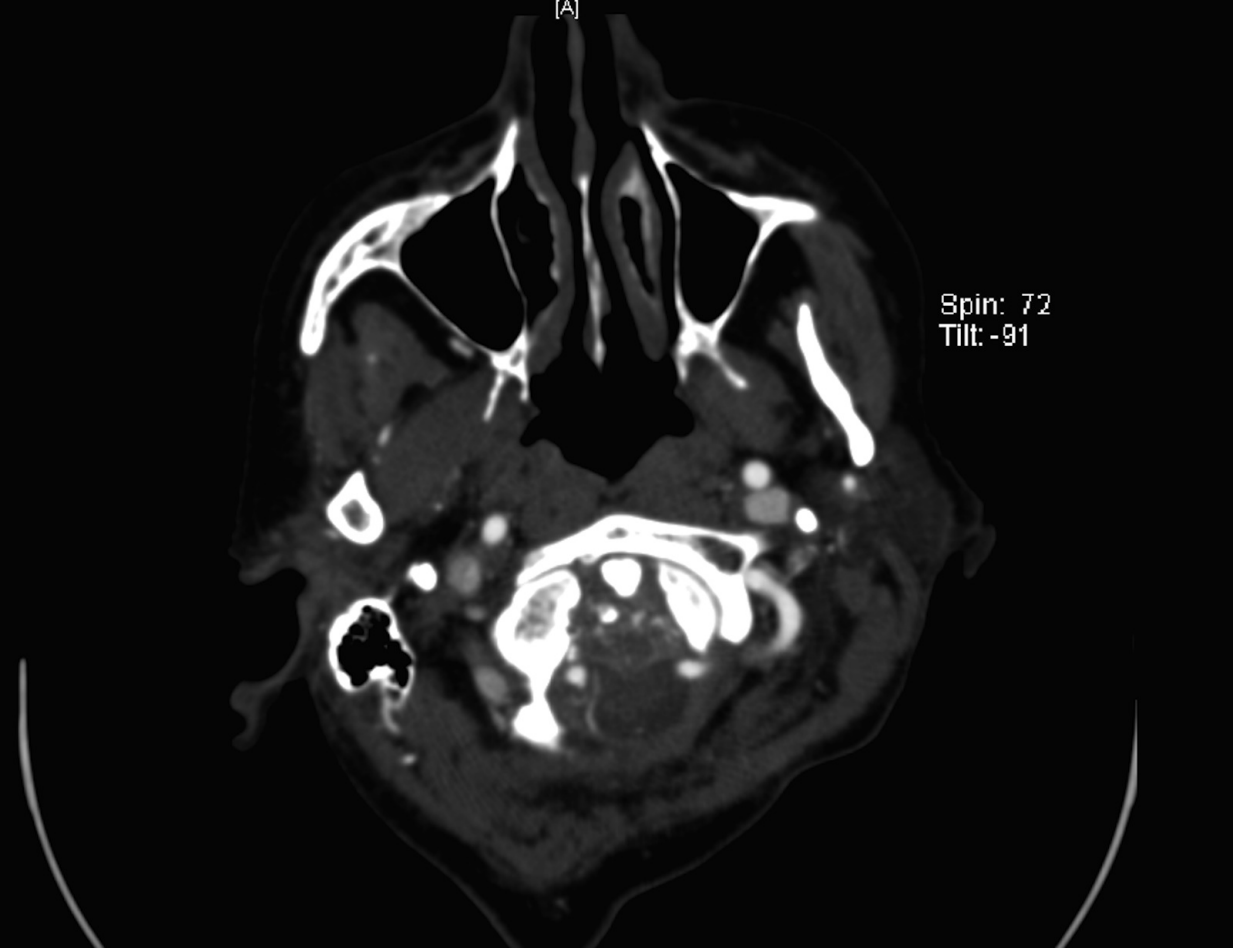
September Axial CT Retrodental mass
